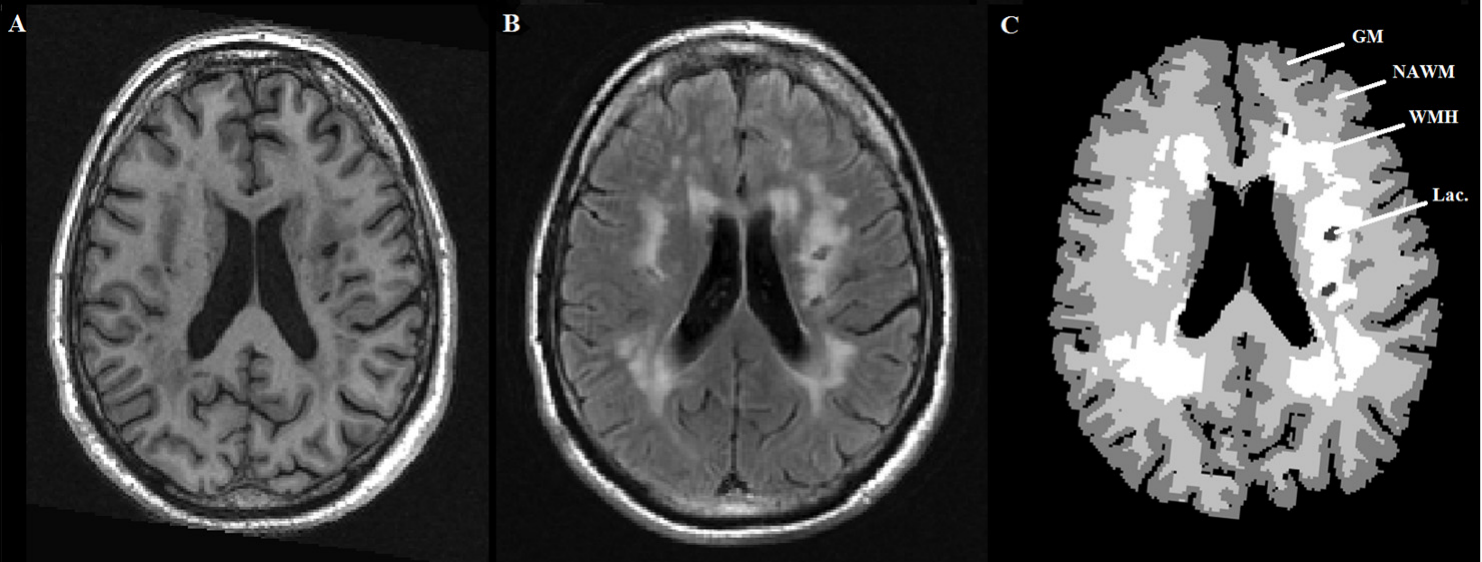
A. T1-weighted image B. FLAIR image C. Corresponding tissue segmentation map. Abbreviations: GM = grey matter; NAWM = normal appearing white matter; Lac. = lacune of presumed vascular origin; WMH = white matter hyperintensity.
